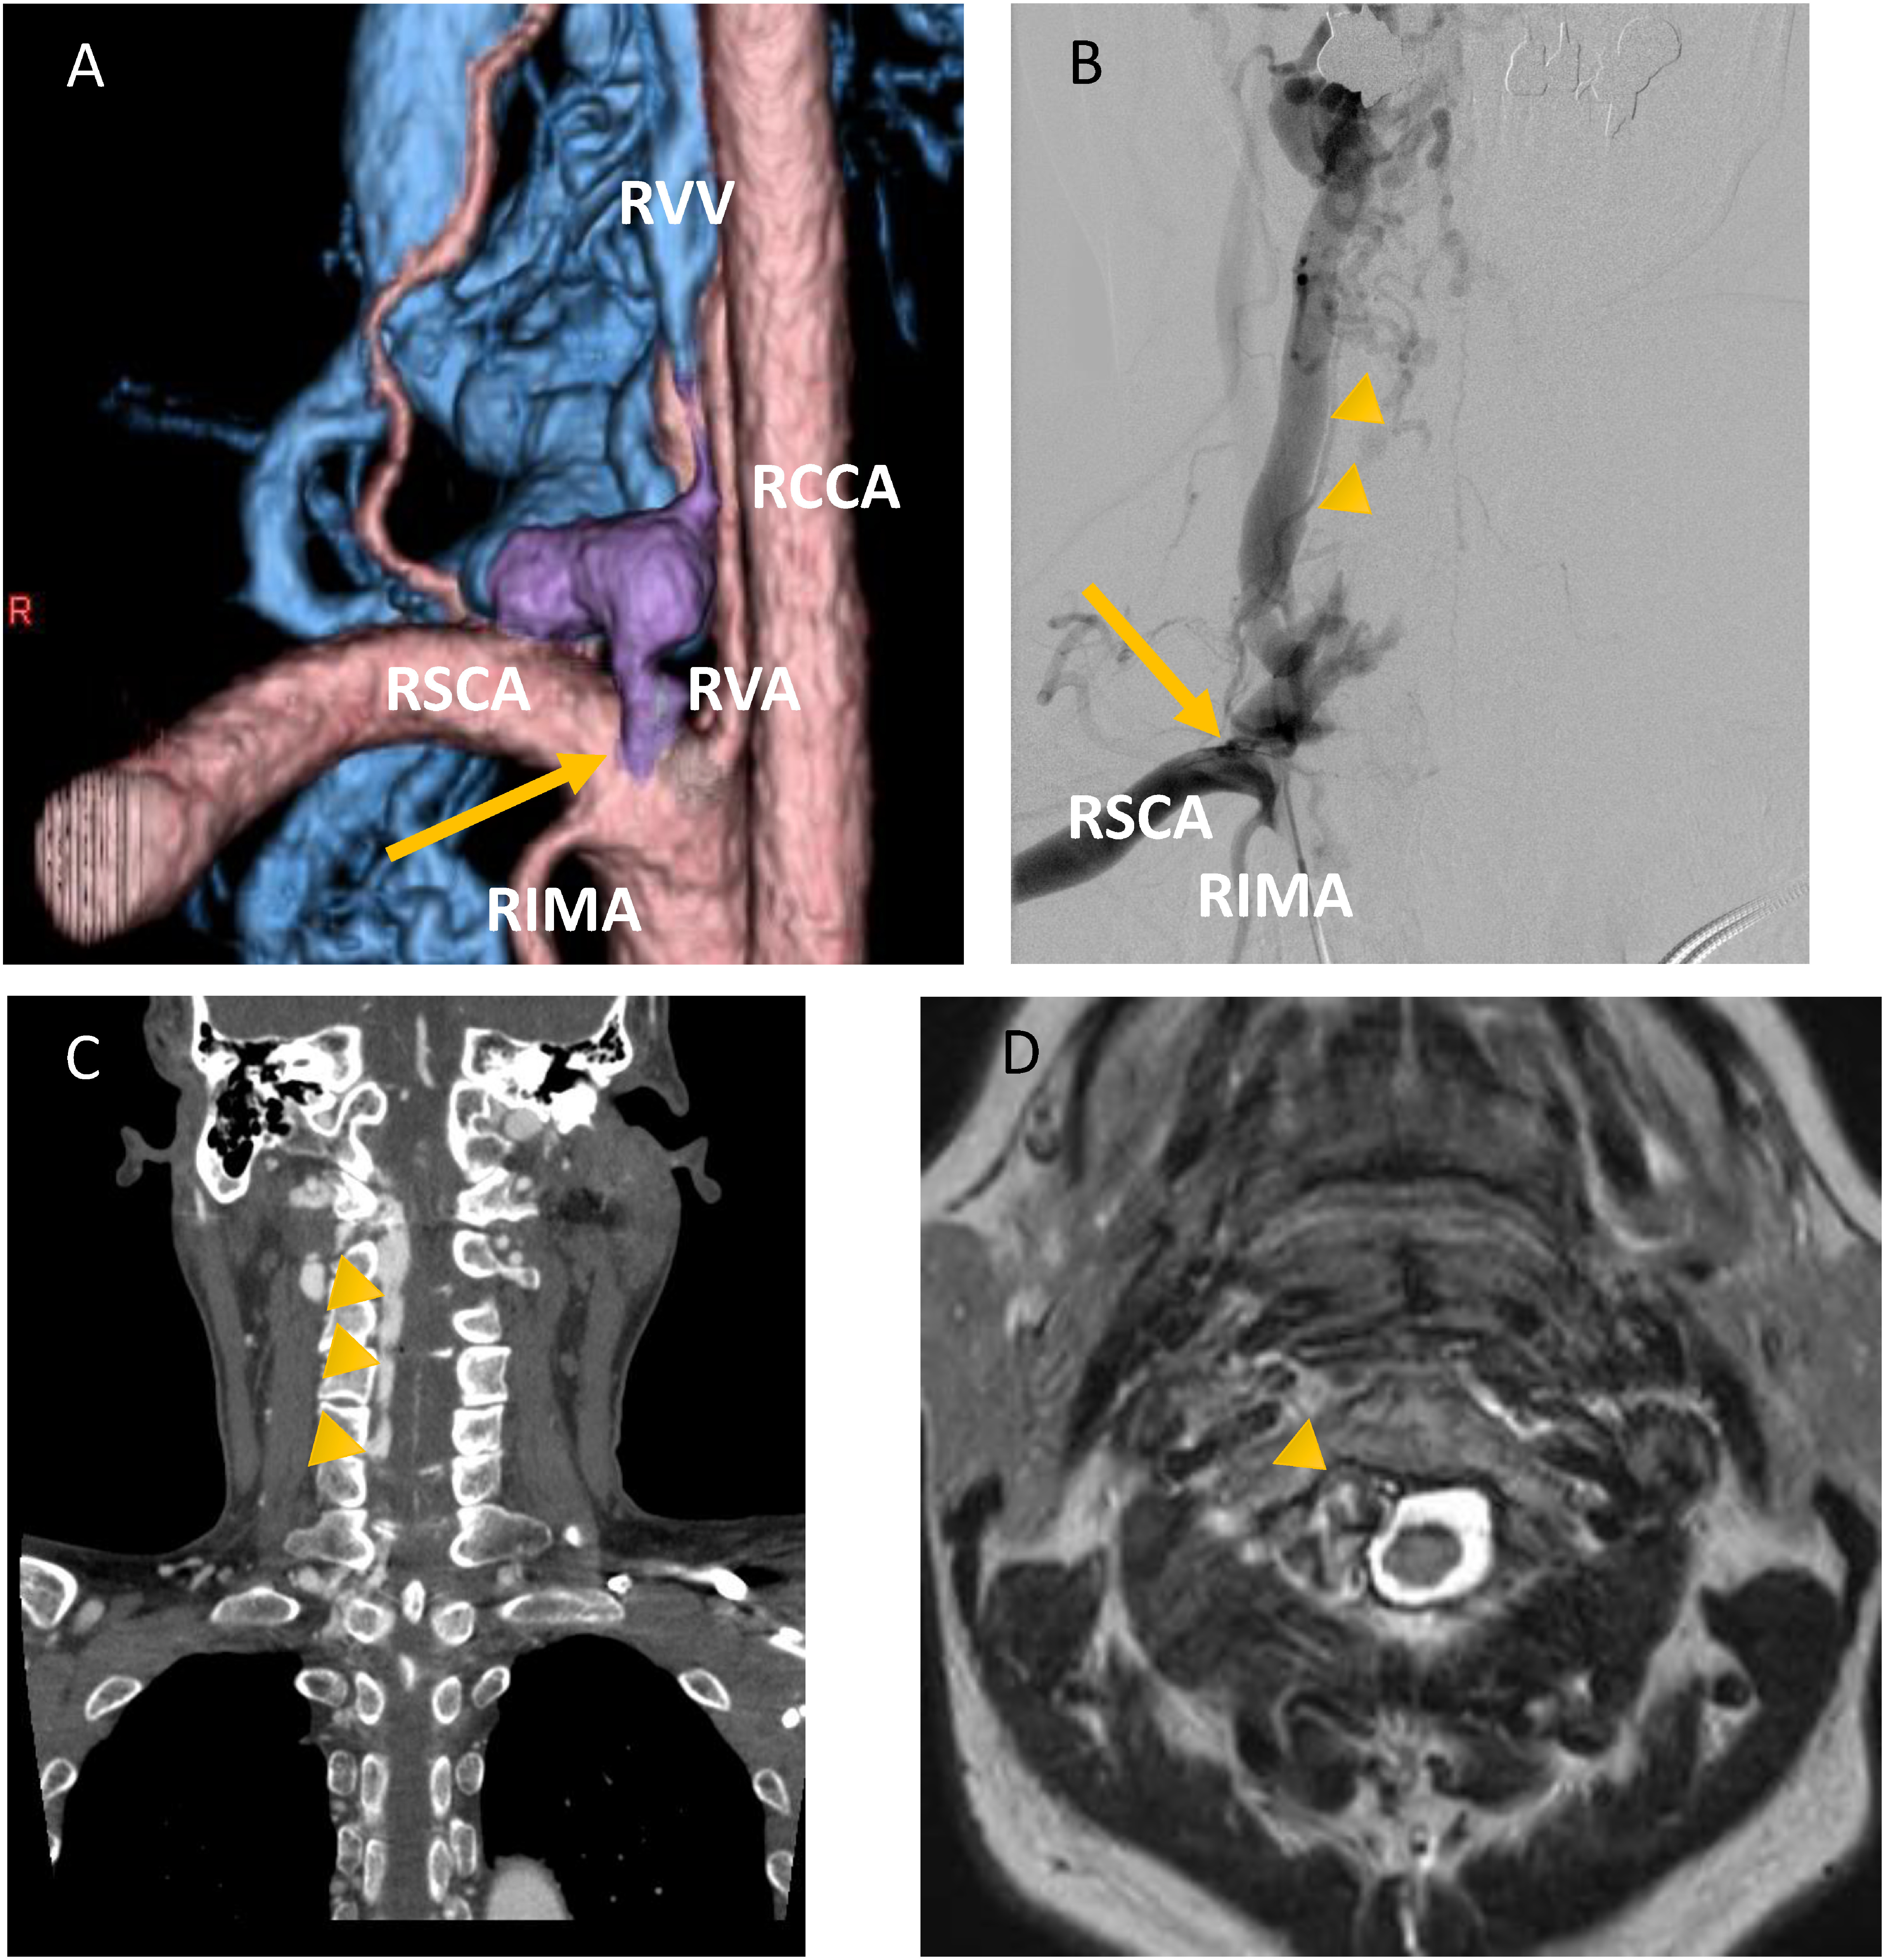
Fig. 1 Arteriovenous fistula and resultant epidural venous dilatation in preoperative computed tomography (A and C), angiography (B), and magnetic resonance imaging (D). The fistula was found between the right subclavian artery and the right vertebral vein (arrow, A and B). Epidural veins were dilated and compressed the spinal cord (arrowheads, B–D).
